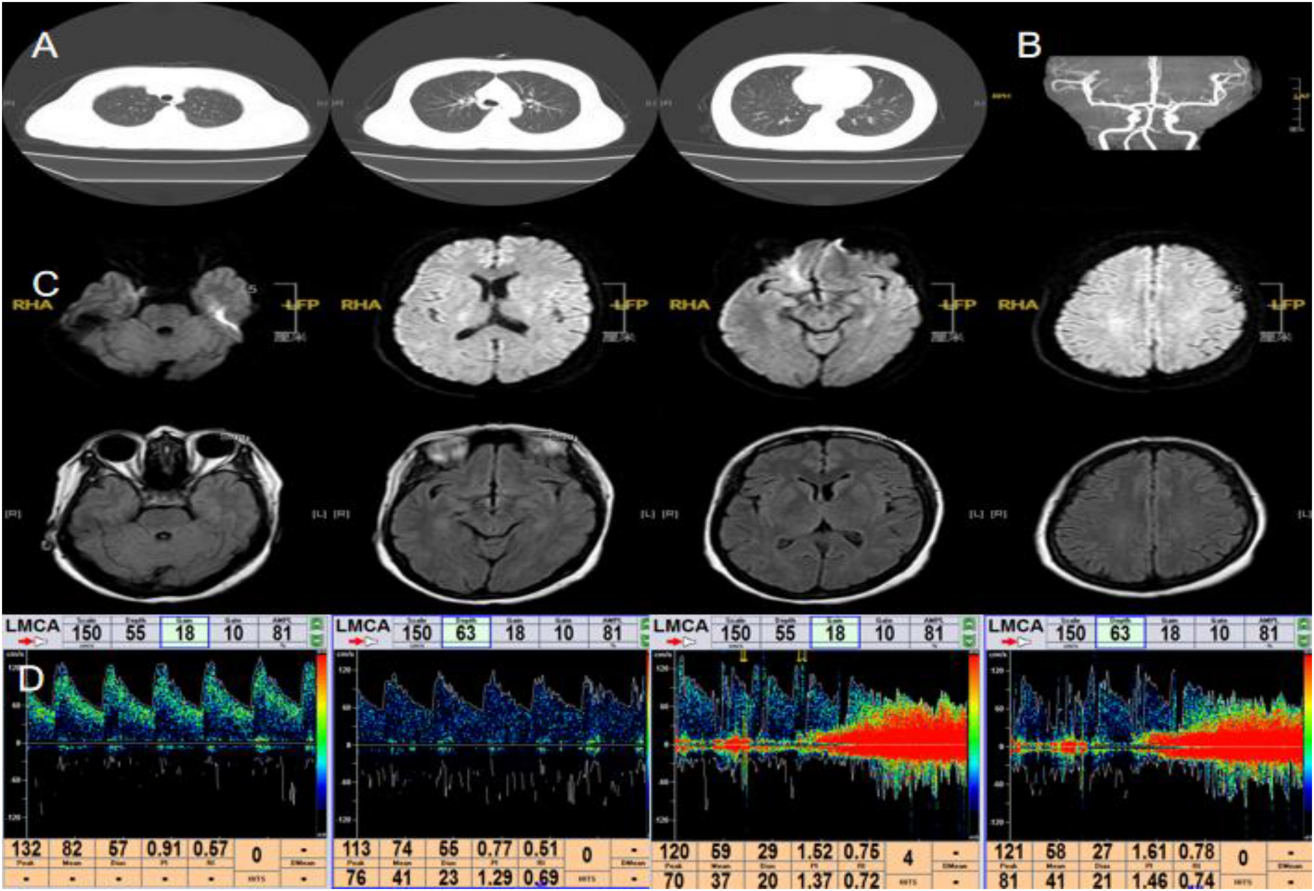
Main examination findings for case 4: (A) chest CT; (B) cranial MRA; (C) cranial MRI; (D) transcranial Doppler ultrasound bubble test.
